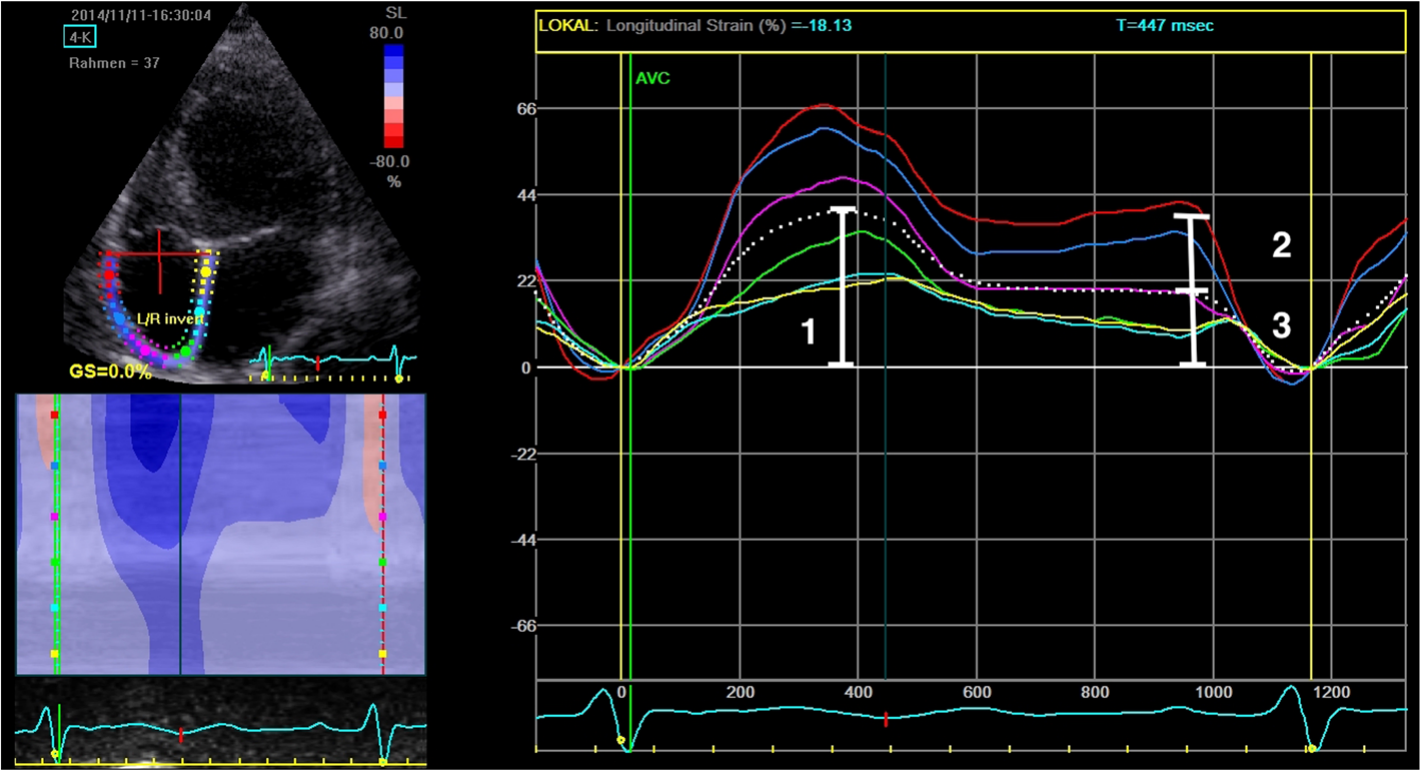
Representative example of speckle tracking echocardiography (2D STE-) derived longitudinal strain of the right atrium obtained in the apical 4-chamber view. Dotted white curve indicates mean of all segments. The maximum excursion of the average strain curve [1] symbolizes the maximum longitudinal strain and RA reservoir function (RAS). RA conduit function was calculated from the difference between RAS and the end of the conduit phase in early diastole [2] and RA contractile function was derived from the difference between the endpoint of conduit phase and the peak RA contraction [3]
